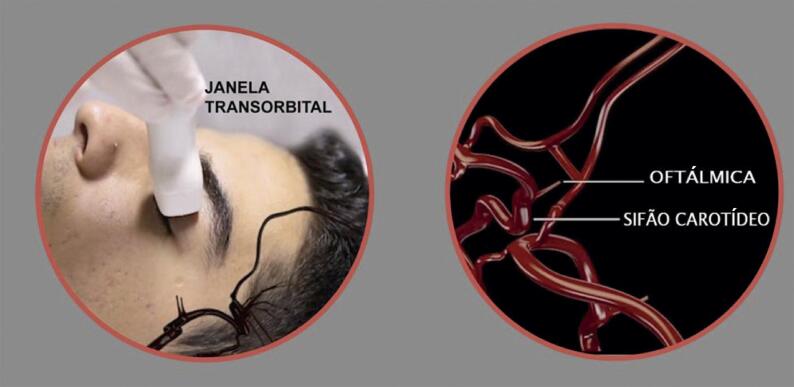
Doppler transcraniano e janela ultrassonográfica transorbital (10A) e representação esquemática das artérias insonadas (sifão carotídeo e oftálmica – 10B).b) Janelas transtemporais:
